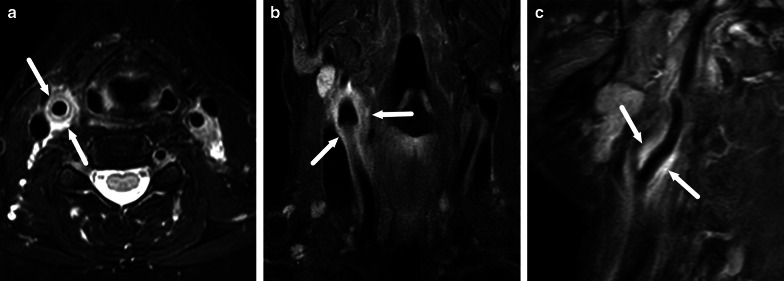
Carotidynia (TIPIC syndrome). Axial fat-suppressed T2-weighted (a) and coronal (b) and sagittal (c) fat-suppressed post-contrast T1-weighted images show inflammation in and around the right carotid sheath
